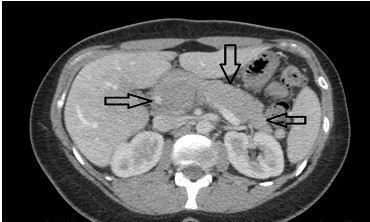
Axial image of abdominal/pelvis computed tomography with contrast demonstrates the thrombus, indicated by the black arrows, in the superior mesenteric vein and extending toward the splenic vein.
